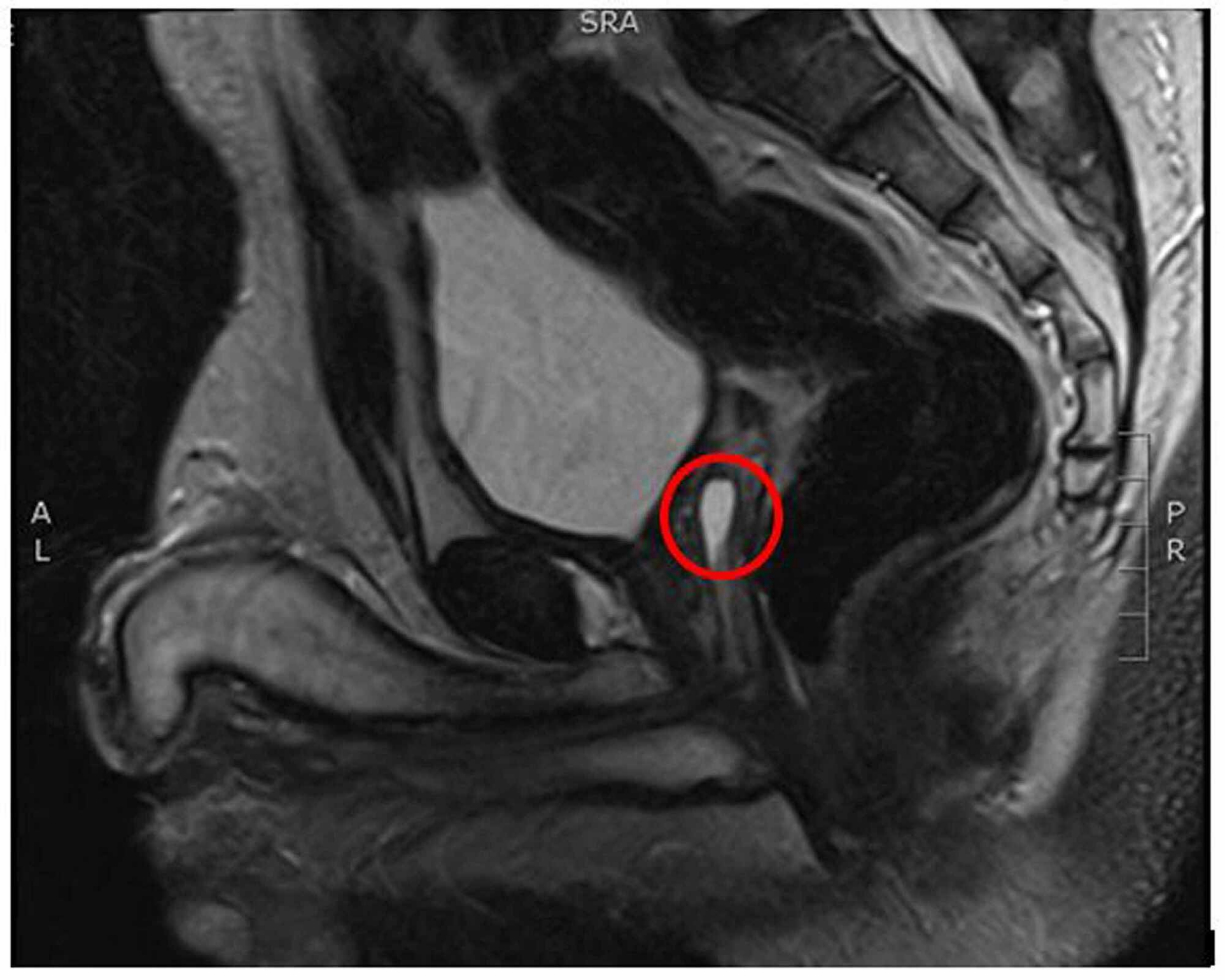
MRI abdomen and pelvis (red circle shows Mullerian duct remnant).
